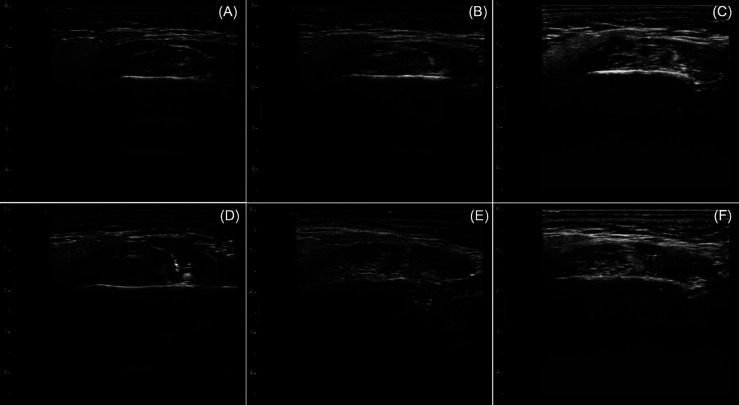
Ultrasonographic captions of the right (A, B, and C) and left (D, E, and F) masseter muscles in axial plane standardized at 2 cm above the mandibular border before treatment (A and D), at 2 weeks after treatment (B and E) and at 4 weeks after treatment. Images show a decrease in muscle thickness, and a change in the intramuscular ultrasonographic appearance.
